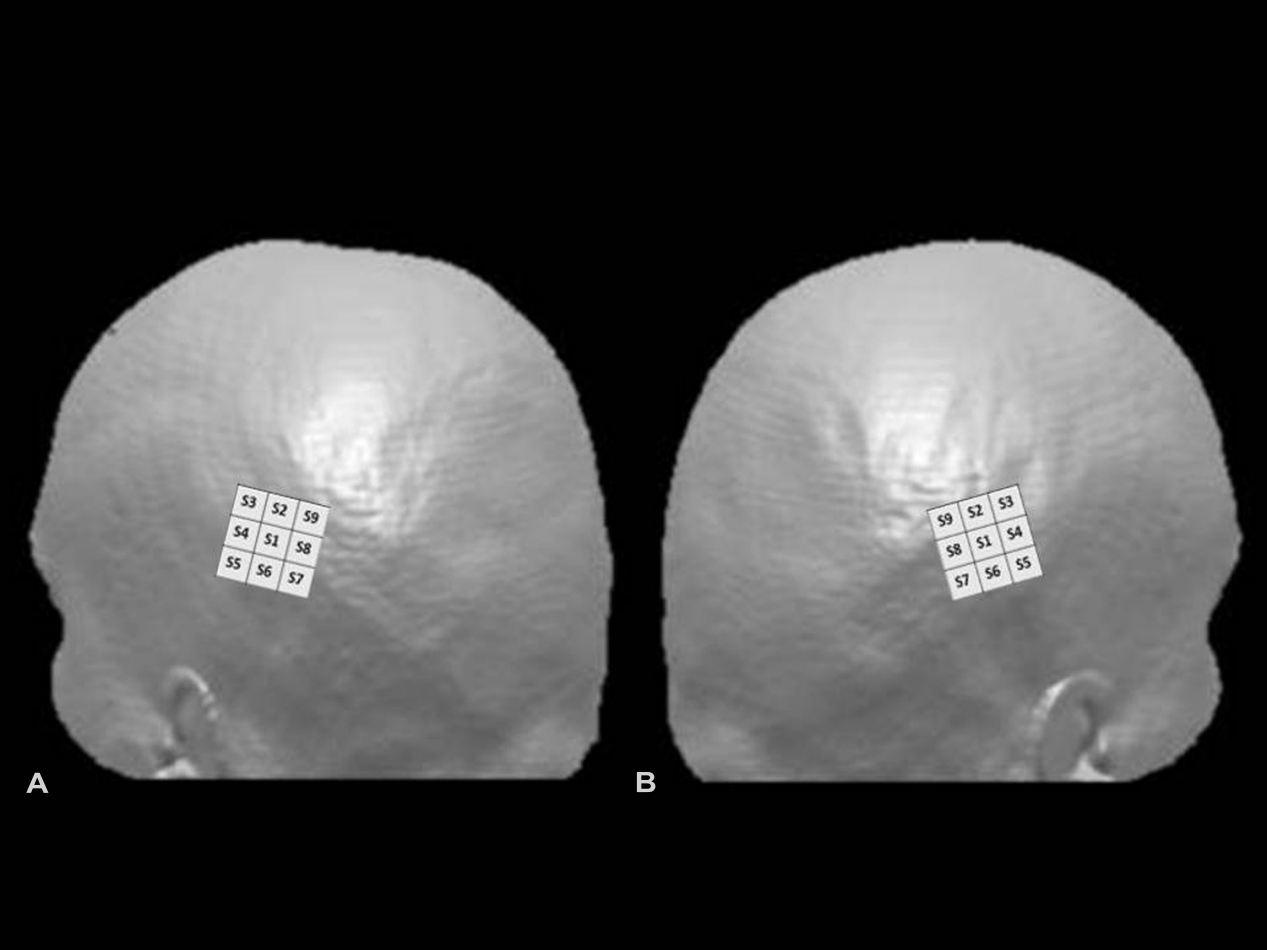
Hunting grids for single-pulse TMS over left (A) and right (B) posterior parietal cortex (PPC). Representation of the grid superimposed on 3D surface rendered MRI of the head using MRIcro (http://www.mricro.com, Rorden and Brett, 2000). In the figure, the 3 cm × 3 cm grid was centered over P3 (left PPC) or P4 (right PPC), according to the 10–20 EEG system. The S7–S8–S9 spots corresponded in both cases to the most posterior and S3–S4–S5 to the most anterior portions of the grid.
